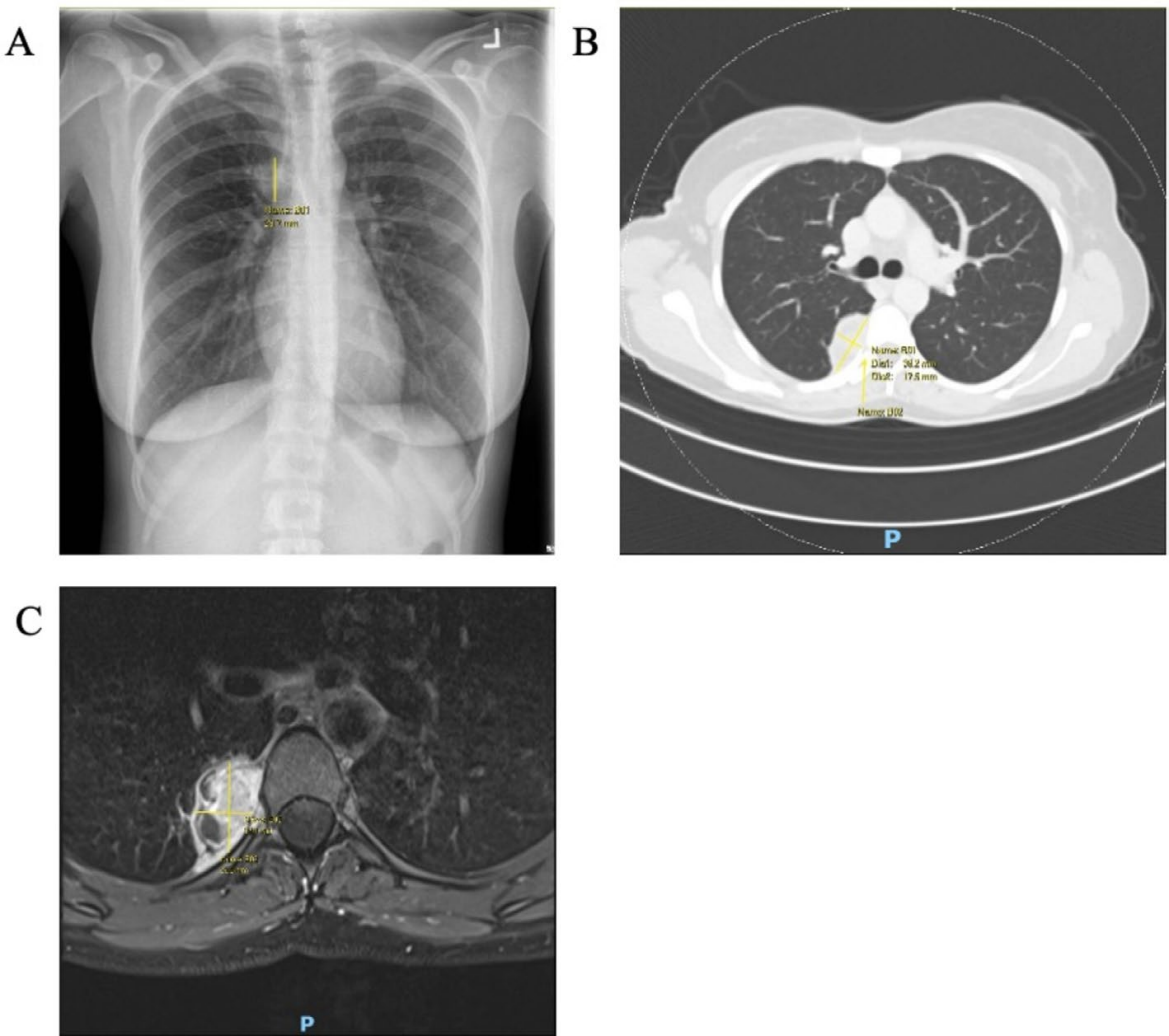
(A) Chest X‐ray showing right upper lobe mass. (B) Contrast‐enhanced CT thorax demonstrating right pleural‐based posterior mediastinal lesion at T5–T6, causing mild rib scalloping without neural foramen extension. (C) Preoperative MRI chest showing multilobulated pleural‐based paraspinal mass at T5–T6 with heterogeneous enhancement and no intraspinal extension.
